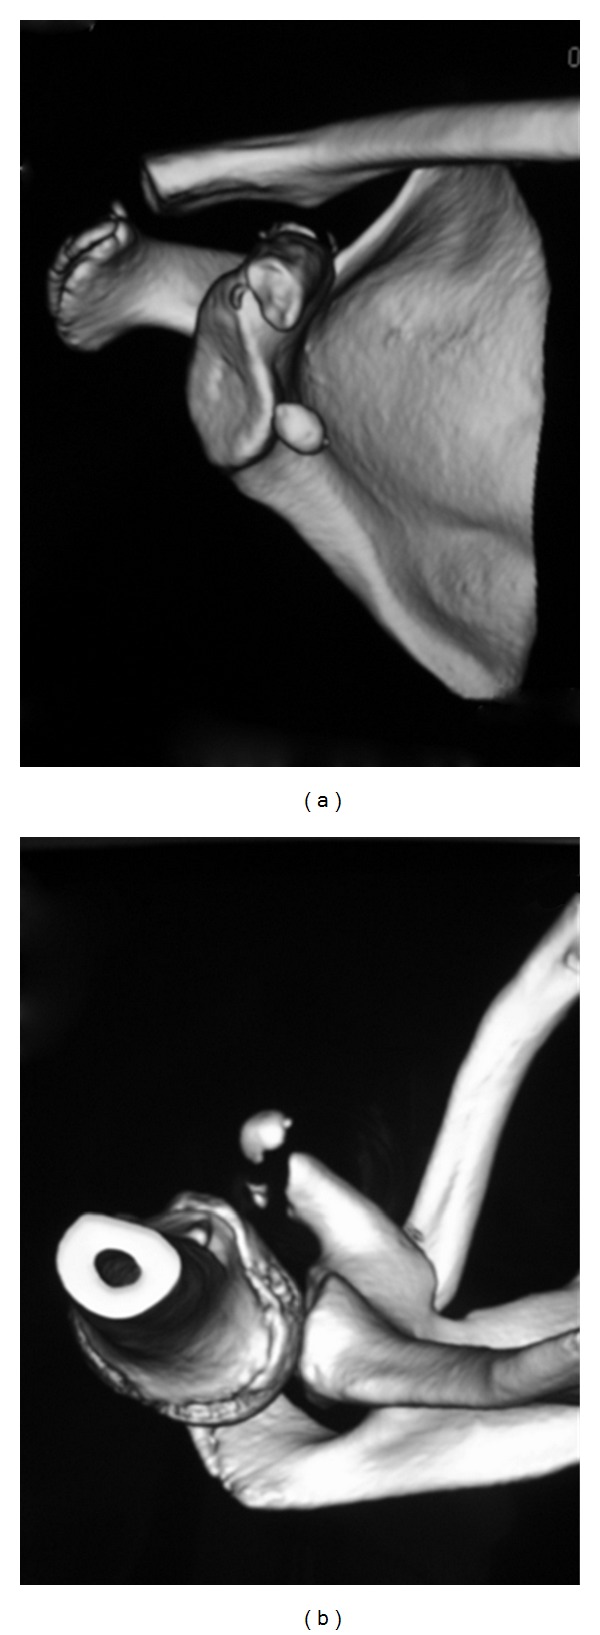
Computed tomography showing two bone fragments resulting from epiphyseal fracture at the origin of the conjoined tendon on the coracoid process. (a) Anteroposterior view. (b) Axillary view.
